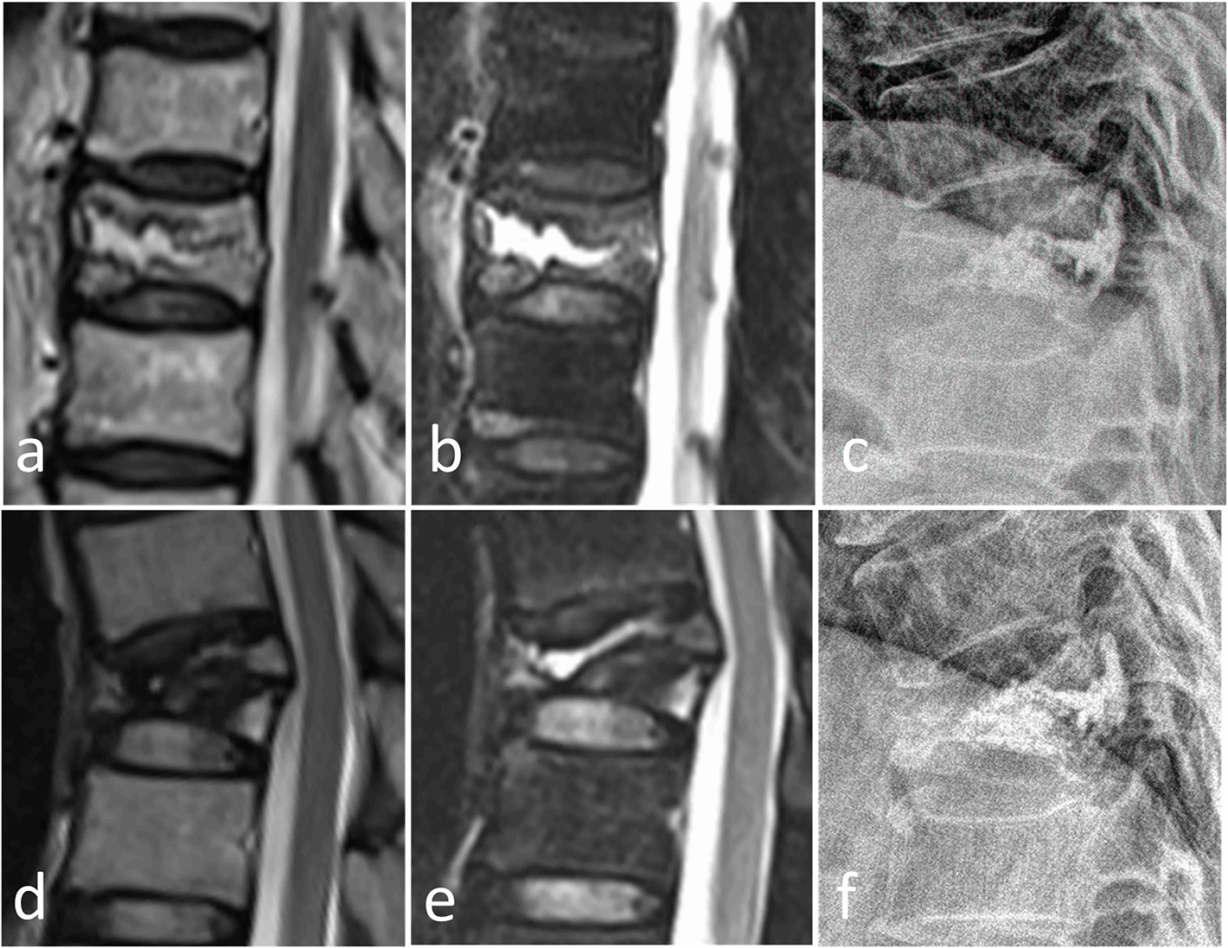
A 59-year-old man with refracture after PVP. Preoperative T2W and SPAIR images (a, b) showed a T10 compression fracture with IVC. Postoperative lateral radiographs (c) after 2 days showed NPEC on the lower and upper endplates. The T2WI, SPAIR image, and lateral radiograph 5 months after PVP (d-f) showed refracture of the cemented T10 vertebra with loss of height and bone marrow edema
